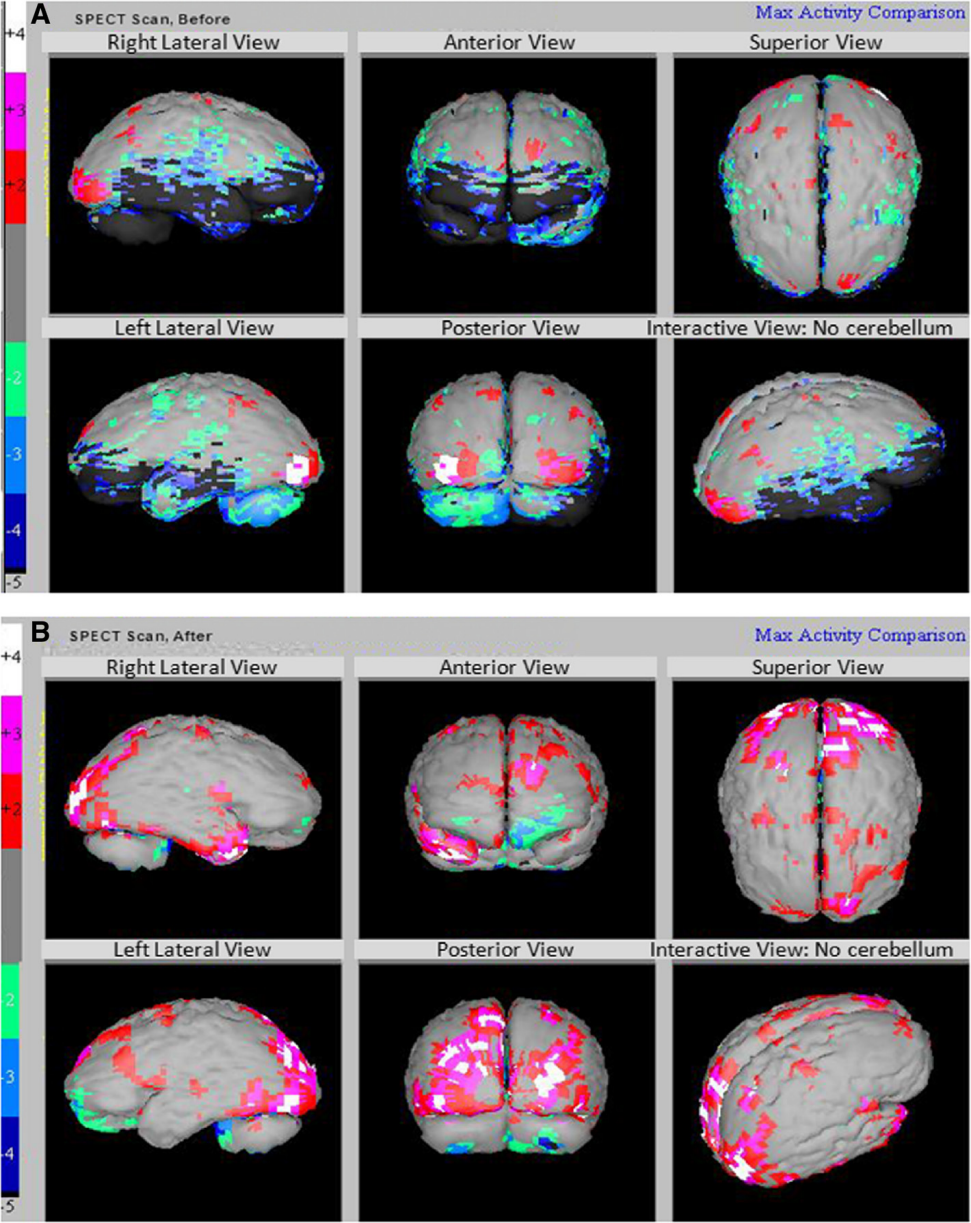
SPECT scan of a cerebral palsy patient (grey - normal; red, pink and white - above normal; green, light/dark blue, black - hypoperfused). A) Showing hypoperfused Regions before Receiving hESC Therapy. B) Improved Perfusion (reduced black areas) after Receiving hESC Therapy.
